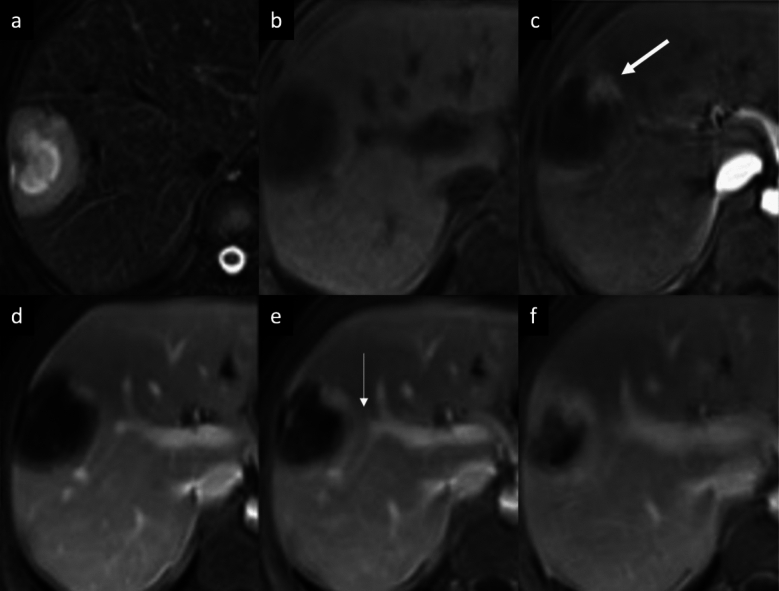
Hepatic epithelioid hemangioendothelioma in a 38 year-old woman. a Axial fat-suppressed T2-weighted MRI shows a subcapsular focal liver lesion in the right lobe with a three-layered target appearance. The high signal intensity of the lesion’s core is surrounded by a thin hypointense ring with a peripheral slight hyperintense halo. Capsular retraction also could be seen. The lesion shows a low signal intensity with a target appearance also on unenhanced T1-weighted MRI (b), with a central area of low signal intensity, surrounded by a thin slight hyperintense irregular ring with a peripheral hypointense halo. Extracellular contrast agent-enhanced MRI demonstrates a peripheral globular discontinuous enhancement on arterial phase (arrow, c), that minimally increases on portal venous (d) and delayed phases at 3′ (e) and 5′ (f). The typical ‘‘lollipop sign’’ is also visible on contrast-enhanced MRI, with portal veins entering and terminating in the periphery of the lesion (thin arrow, e)
